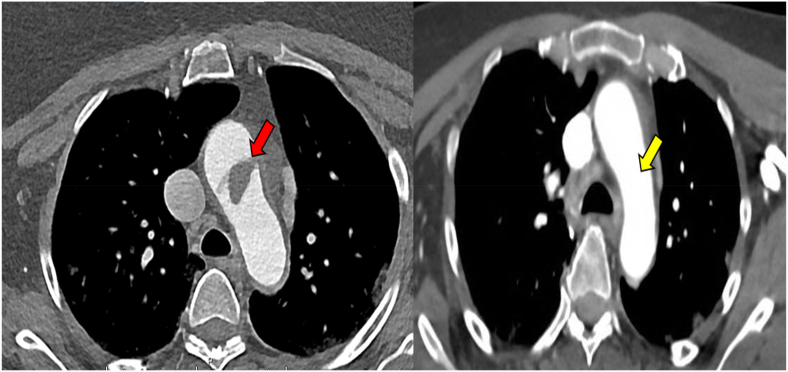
Axial CT scan of the chest with contrast in soft tissue/bone window that shows an aortic arch partial filling defect (red arrow) suggestive of thrombus formation with resolution of the thrombus 10 days later (yellow arrow) after anticoagulant therapy. (For interpretation of the references to colour in this figure legend, the reader is referred to the Web version of this article.)
